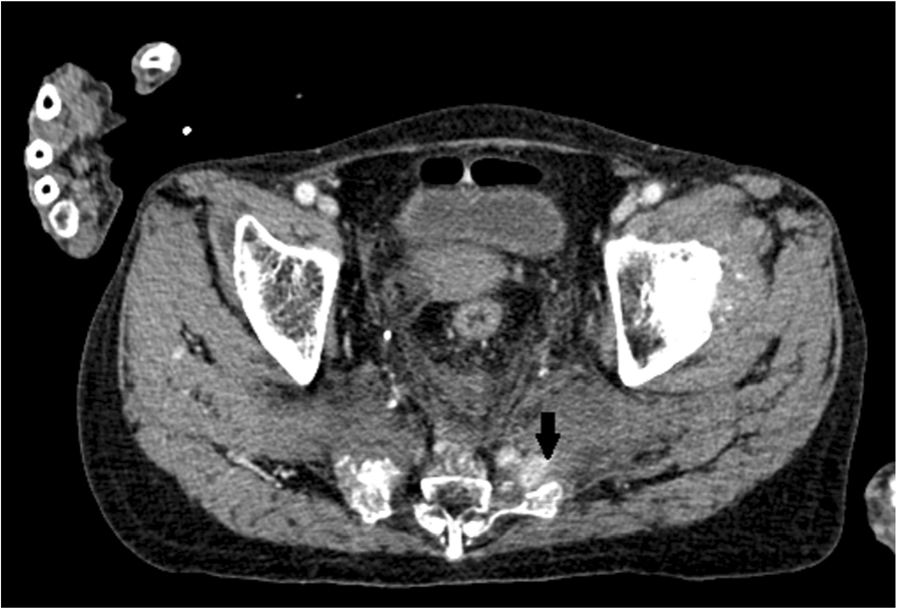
Contrast-enhanced computed tomography of the patient with pelvic fractures after trauma. The *arrow* indicates a hematoma with extravasation
